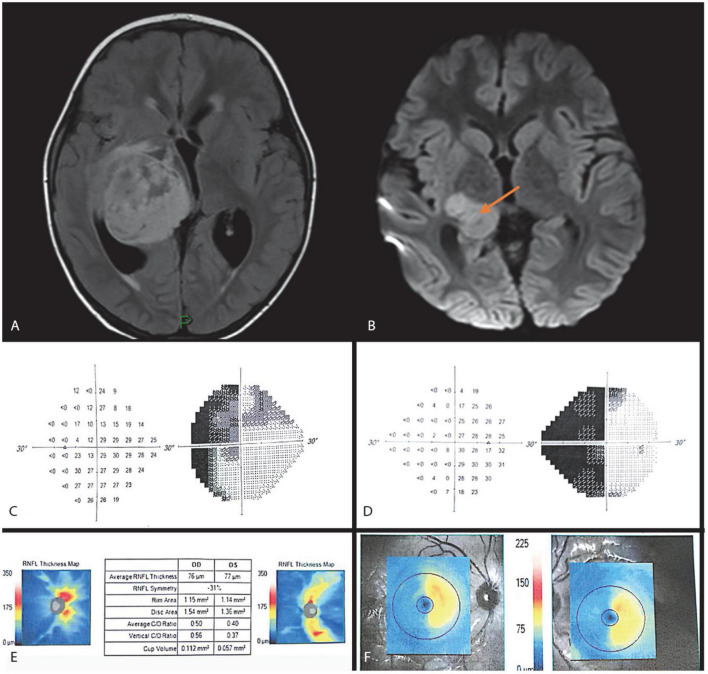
Case 3. MRI FLAIR and DWI images (A,B) show a right thalamic lesion extending to the right optic radiation causing significant mass effect and hydrocephalus. Automated perimetry at 12 months after surgery showing left homonymous hemianopia (C,D). OCT 6 months after surgery showing RNFL thinning in the right superior and inferior and left nasal and temporal quadrants (E), and related retinal ganglion cell counts (F).
